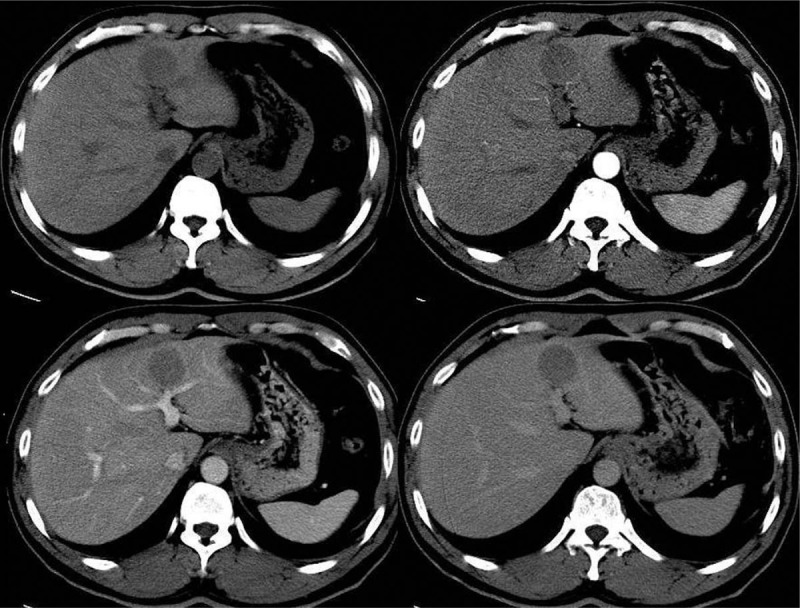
A small round mass in left-lateral lobe, which showed no enhancement in dynamic CT scan.
